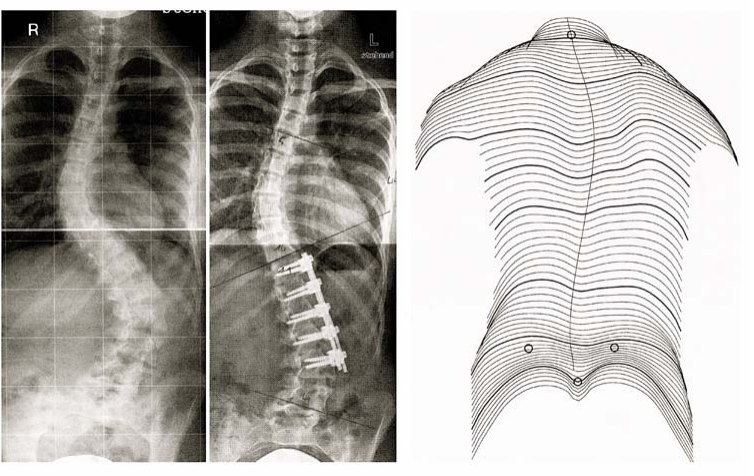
On the left X-rays before and after surgery and on the right clinical appearence after surgery. The patient is unbalanced. Due to the short instrumentation area there is a sharp angle at the junctional lumbo-pelvic area making it reasonable to assume that a reoperation will be necessary some day.
